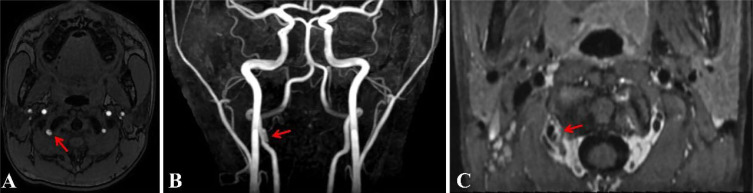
(A-B) A dissecting aneurysm in the right VA (V3 segment); (C) Thrombosis within the false lumen demonstrated on high-resolution MRI.
